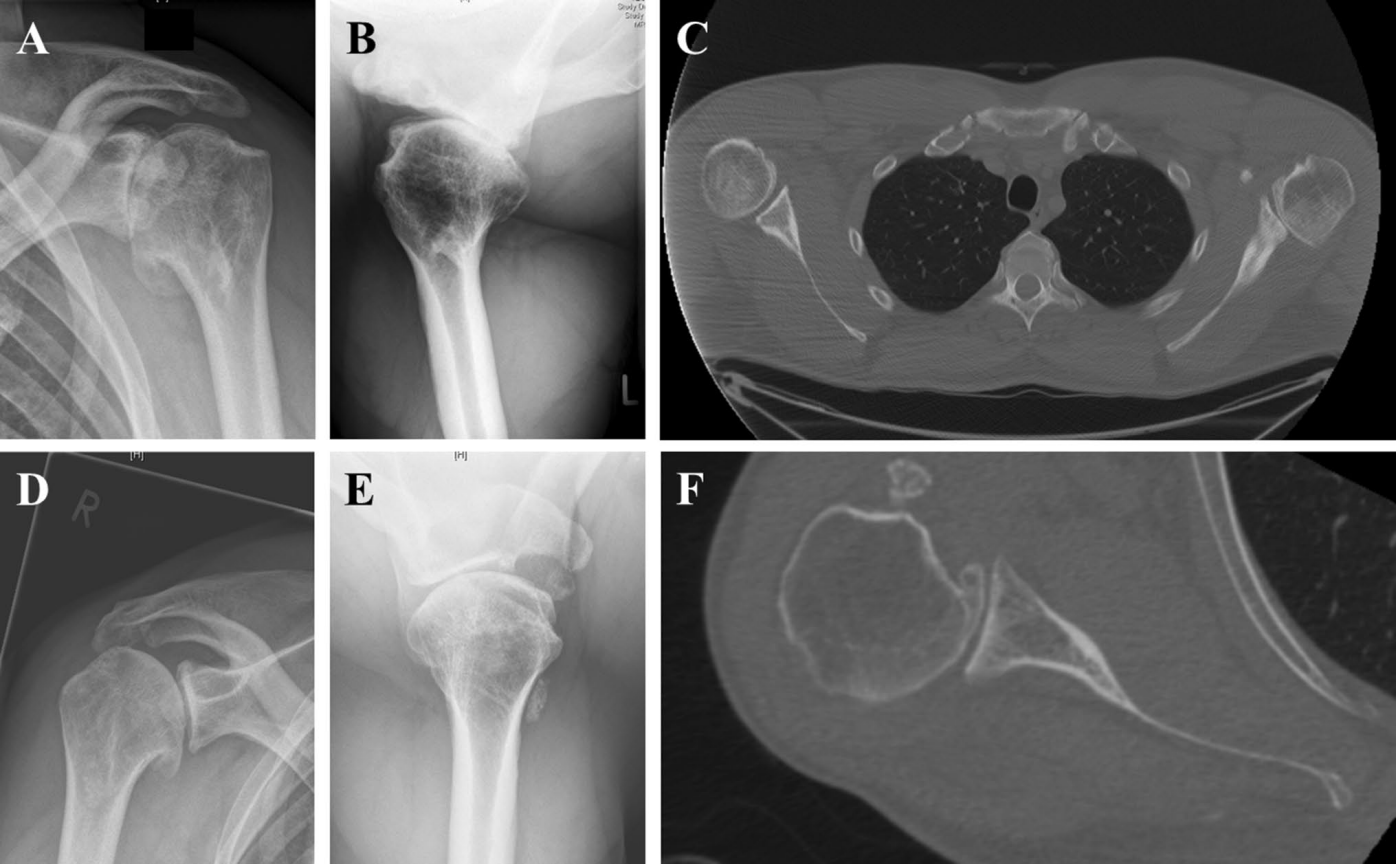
Preoperative radiographics of a 40-year-old male patient. The BMI is 29, he is a current smoker and the shoulder-activity level is 4 with participating in boxing, combat sport and weightlifting. He used to be a professional bodybuilder. a–c Conventional x-rays with a.p. a and axial b images revealing a severe primary glenohumeral osteoarthritis (GOA) of the left shoulder. Computed tomography scan showed a posterior subluxation of the humeral head with posterior glenoid erosion. d–f Three years later, the patient presented with progressive shoulder complaints on the contralateral shoulder. Radiographs revealed a similar posterior eccentric GOA of the right shoulder joint
